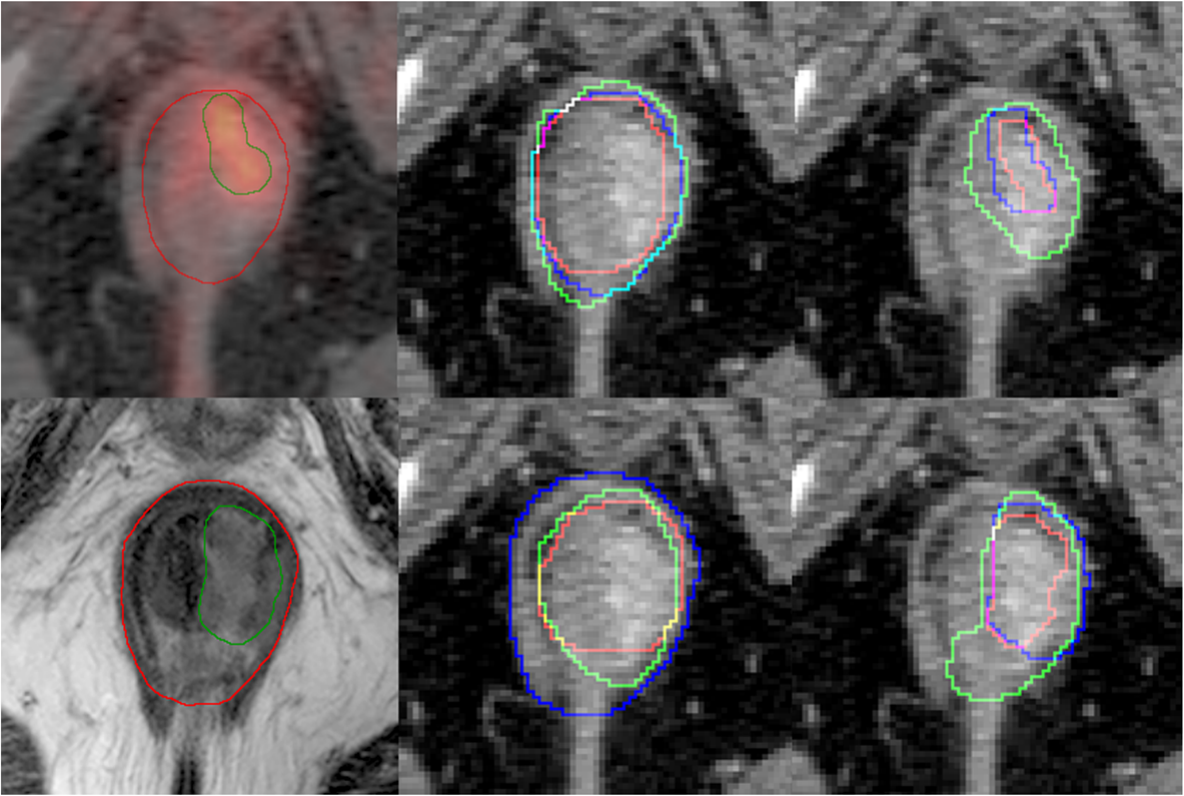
Delineations in one patient; all images from the same slice. Top row shows PET-based delineations. The left image is a single observer delineation of GTV (red) and GTV_T_ (green) in the PET/CT basis. The central image compares the three observers’ GTVs with each other, and the right compares the GTV_T_s. These two latter panels show delineations in the planning CT basis. The bottom row shows three corresponding MRI-based delineations
